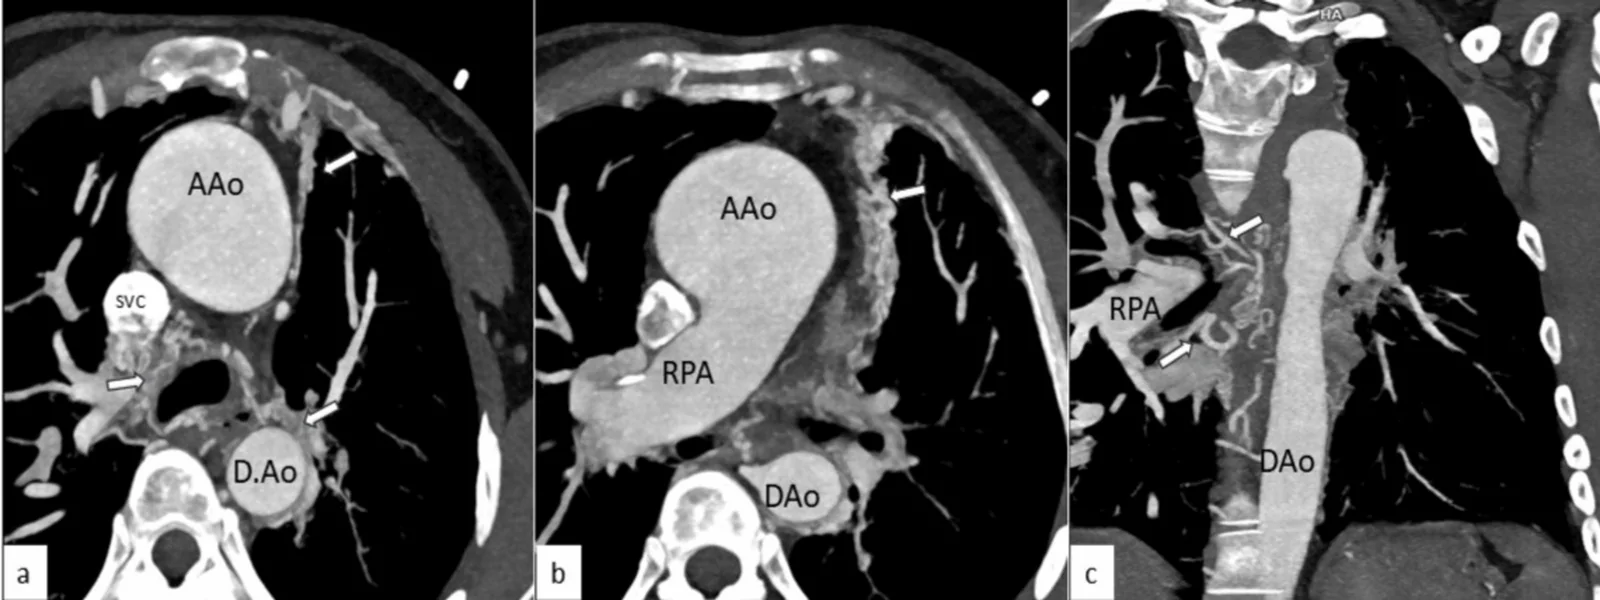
Cardiac CT demonstrating extensive mediastinal collaterals (a to c) Cardiac CT Axial images showing multiple extensive collaterals in the subcarinal, AP window, peribronchial, along the tracheal wall, mediastinal, bilateral hilar, and epicardial surface (block arrows). RPA- right pulmonary artery, AAo- Ascending Aorta, DAo- Descending Aorta, RPA- Right pulmonary artery, SVC- Superior vena cava.
